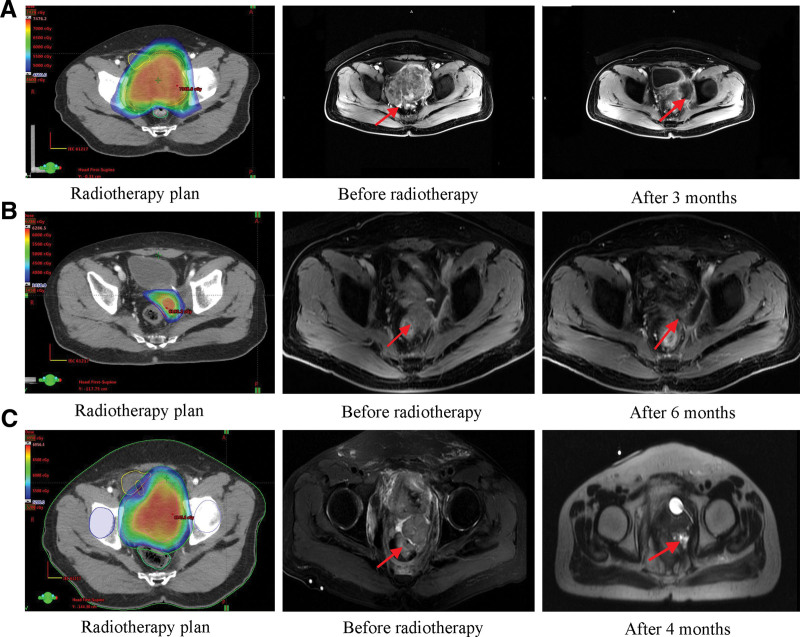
(A) Radiotherapy plan, MRI comparison of primary prostate lesion before radiotherapy and after radiotherapy for 3 months (achieved PR). (B) Radiotherapy plan, MRI comparison of local recurrent pelvic lesion before radiotherapy and after radiotherapy for 6 months (achieved PR). (C) Radiotherapy plan, MRI comparison of third local recurrent pelvic lesion before radiotherapy and after radiotherapy for 4 months (achieved PR). MRI = magnetic resonance imaging, PR = partial response.
